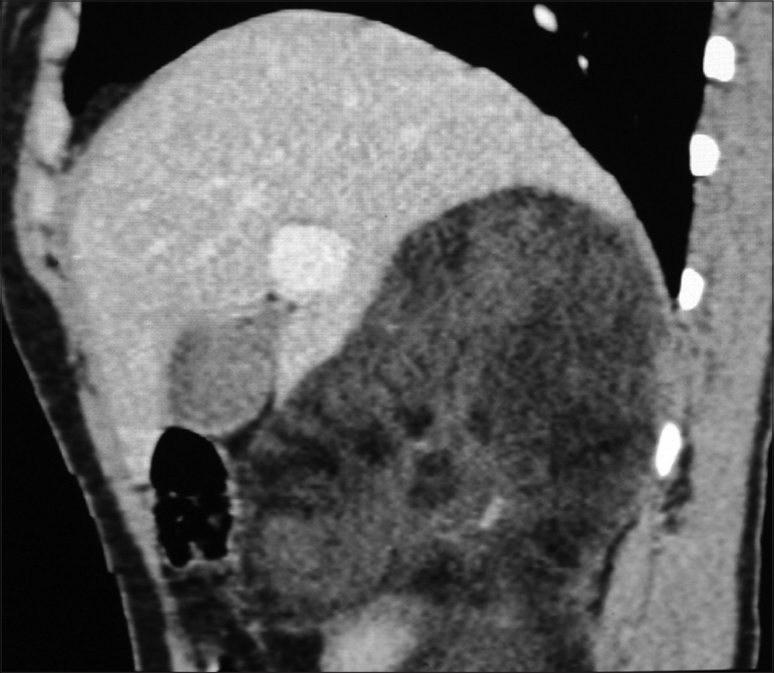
Sagittal reformation CT shows the fat density mass with some more dense components inside, interposed between liver and upper pole of right kidney
